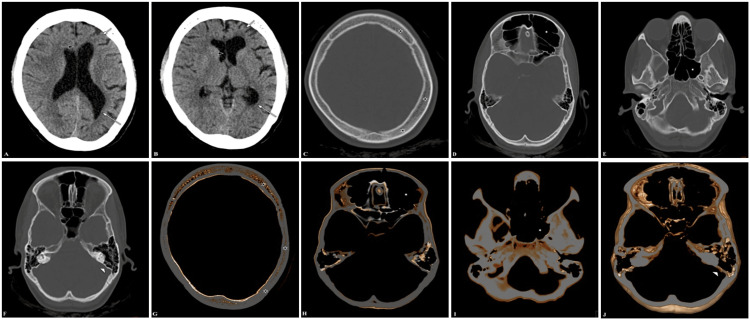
Case presentation 3 - CT brain Axial sections in brain window (A, B) show left hemicerebral atrophy (short white arrow). There is ipsilateral lateral ventricle ex vacuo dilatation (long white arrow) and a minimal midline shift of falx cerebri to the ipsilateral side (short black arrow) at the corona radiata and Sylvian fissure level, respectively. Axial sections in the bone window (C, D, E, F) and 3D axial VR (G, H, I, J) show compensatory ipsilateral calvarial hypertrophy (black asterisks), ipsilateral frontal and sphenoid sinus hyperpneumatization (white asterisk), and ipsilateral mastoid air cell hyperpneumatization (small white arrowhead), respectively. CT: computed tomography; VR: volume rendering
